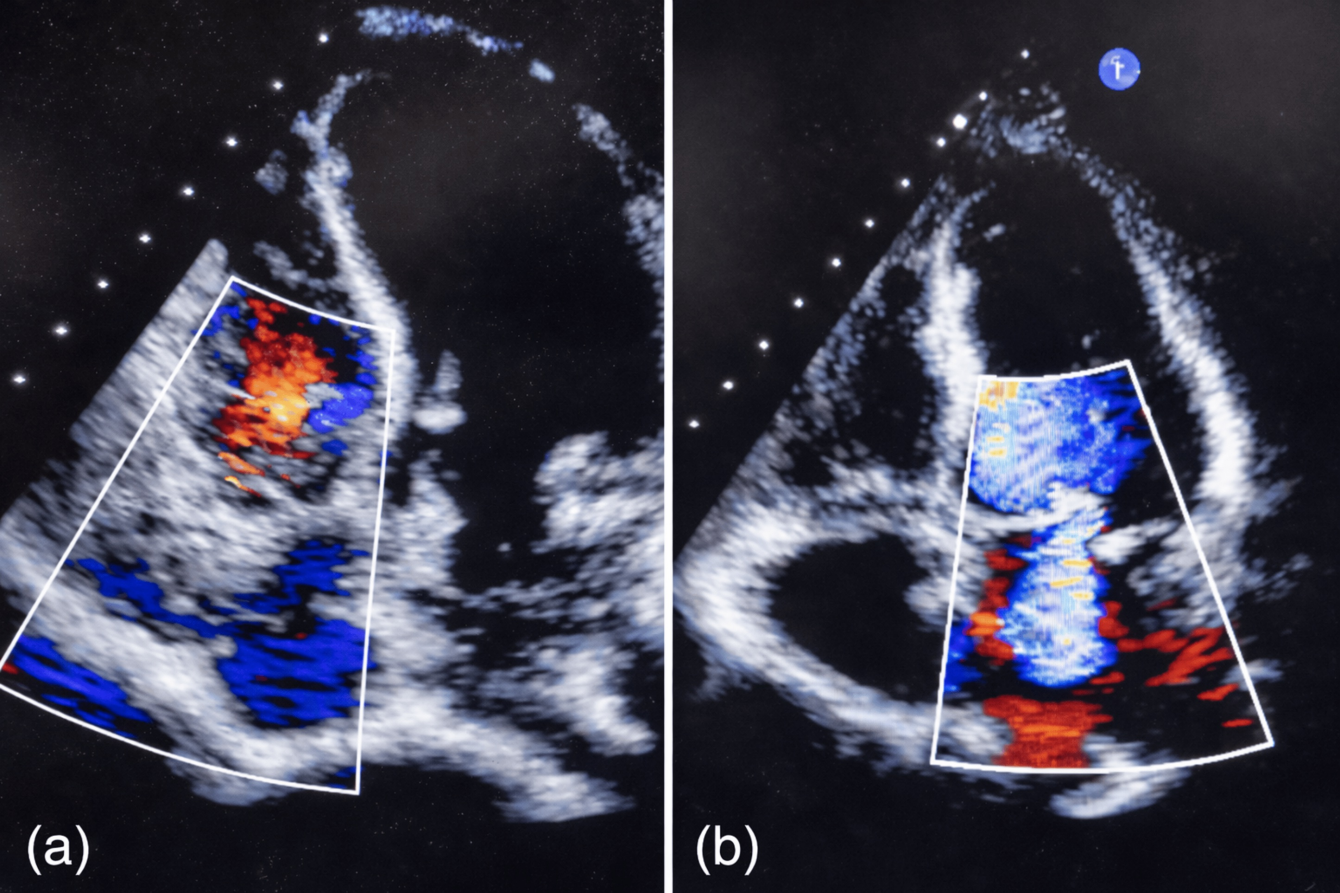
Transthoracic echocardiography with colour doppler imaging demonstrating (a) tricuspid regurgitation and (b) mitral regurgitation, without evidence of valvular vegetations.
